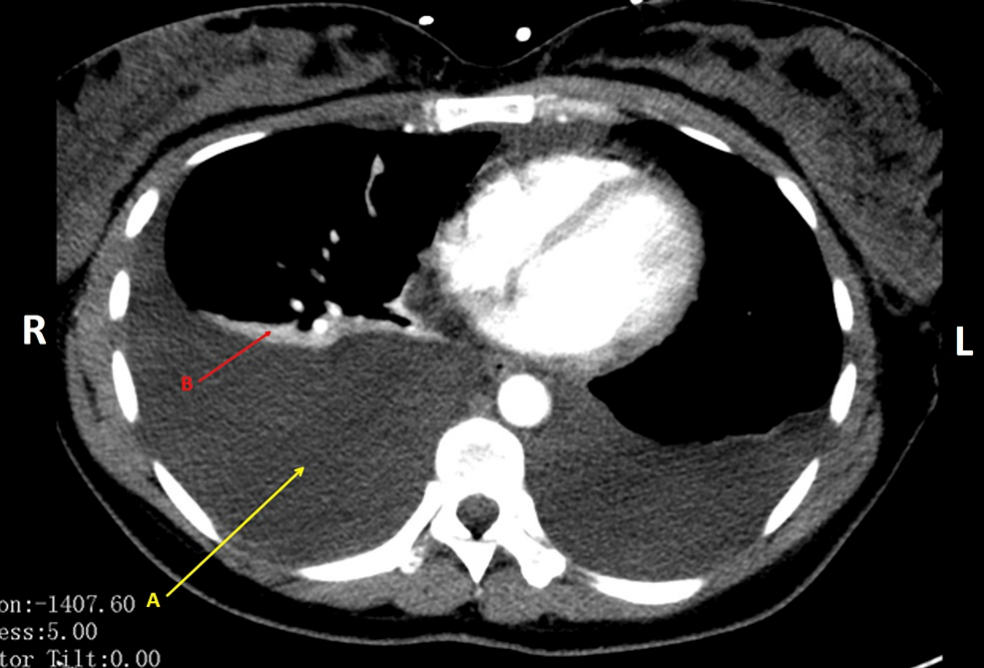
CT imaging of the chest demonstrated a large right pleural effusion and a moderate left pleural effusion. Note the moderate right pleural effusion (A) with associated atelectasis (B).
